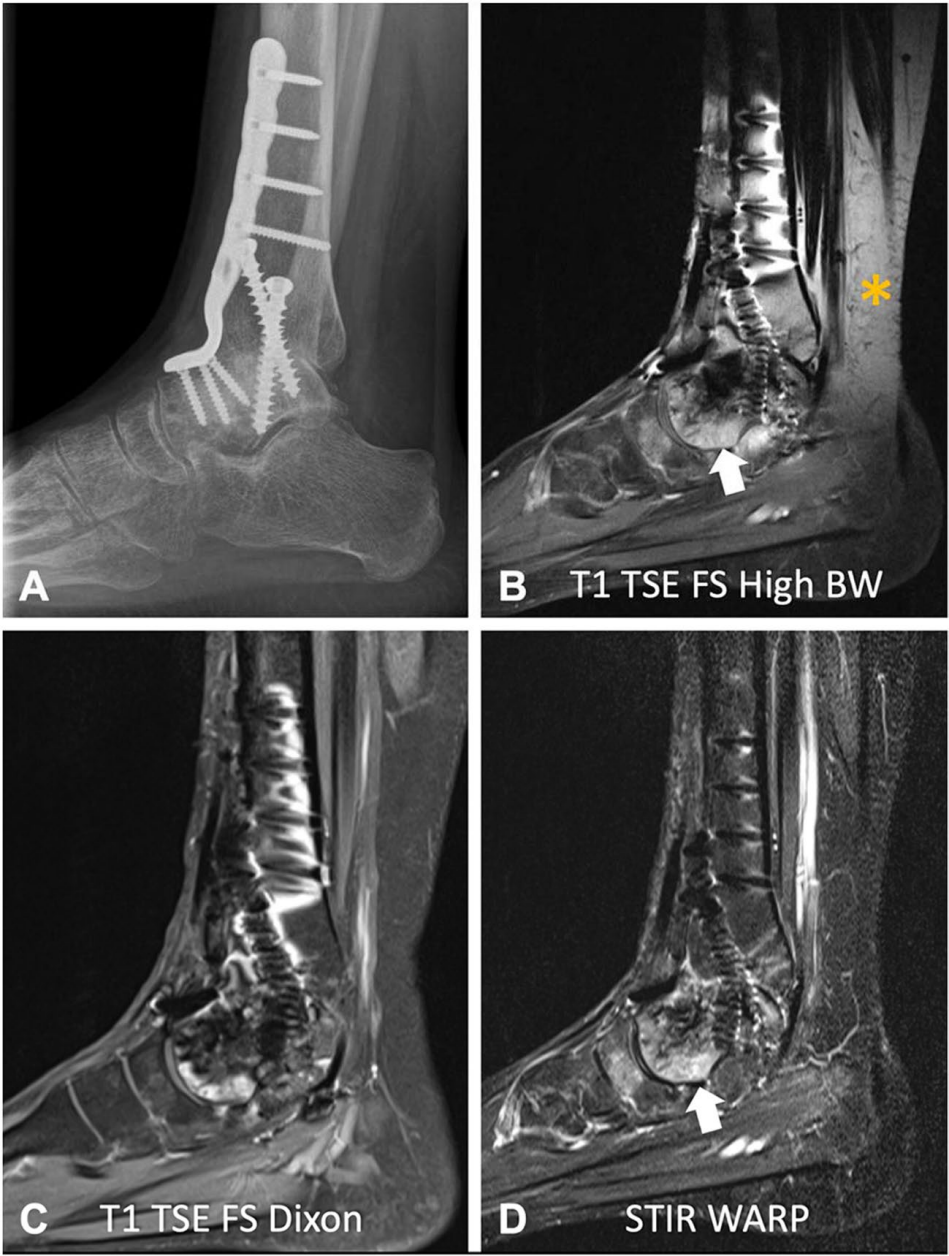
A 54-year-old patient after arthrodesis of the upper ankle joint following curettage and plombage of an aneurysmatic bone cyst with titanium screws and plates. The patient suffered from recurrent ankle pain on movement. The lateral radiograph (A) shows several types of metal implants in different orientations. The high bandwidth (BW) sagittal T1-weighted fat-suppressed turbo spin echo (TSE) sequence (B, bandwidth 372 Hz/pixel) shows severe signal accumulation and geometric distortion with failure of fat saturation (asterisk). Note also that the failure of fat suppression does not allow a reliable detection of the bone marrow edema in the talus (arrow), which was most likely the source of the ankle pain, as the signal in the talus looks similar to the signal in the distal tibia. The sagittal T1-weighted Dixon sequence (C) provides adequate overall fat suppression, but significant focal artifacts remain around the implants. A sagittal short tau inversion recovery (STIR) turbo spin echo sequence (D) with optimized inversion pulse (STIR WARP) demonstrated the least artifacts and provided adequate fat suppression, visualizing the bone marrow edema of the talus head (arrow). The images were acquired at 1.5 T with following sequence parameters: T1 TSE FS High BW—echo time (TE) 10 ms, repetition time (TR) 631 ms, receiver bandwidth 372 Hz/pixel; T1 TSE FS Dixon—TE 12 ms, TR 655 ms, receiver bandwidth 248 Hz/pixel; STIR WARP—TE 48 ms, TR 4000 ms, receiver bandwidth 385 Hz/pixel, inversion time (IT) 160 ms
